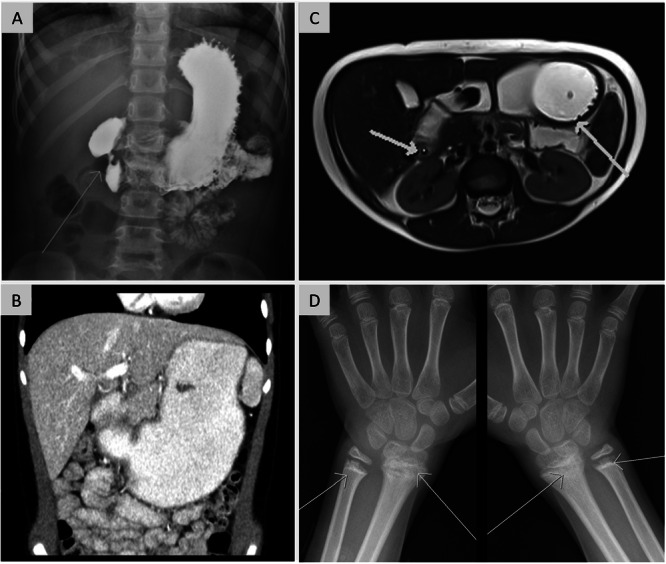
Radiographic investigations. (A) Upper GI barium study showing marked narrowing in the second part of the duodenum (arrow) and mucosal fold thickening in the stomach. (B) Coronal CT image showing a large stomach and wall thickening in the proximal duodenum. (C) Axial HASTE MR image showing wall thickening in the duodenum (short arrow) and gastric fundus (long arrow). (D) Hand x‐ray showing metaphyseal irregularity and sclerosis in bilateral distal radius and ulna.
